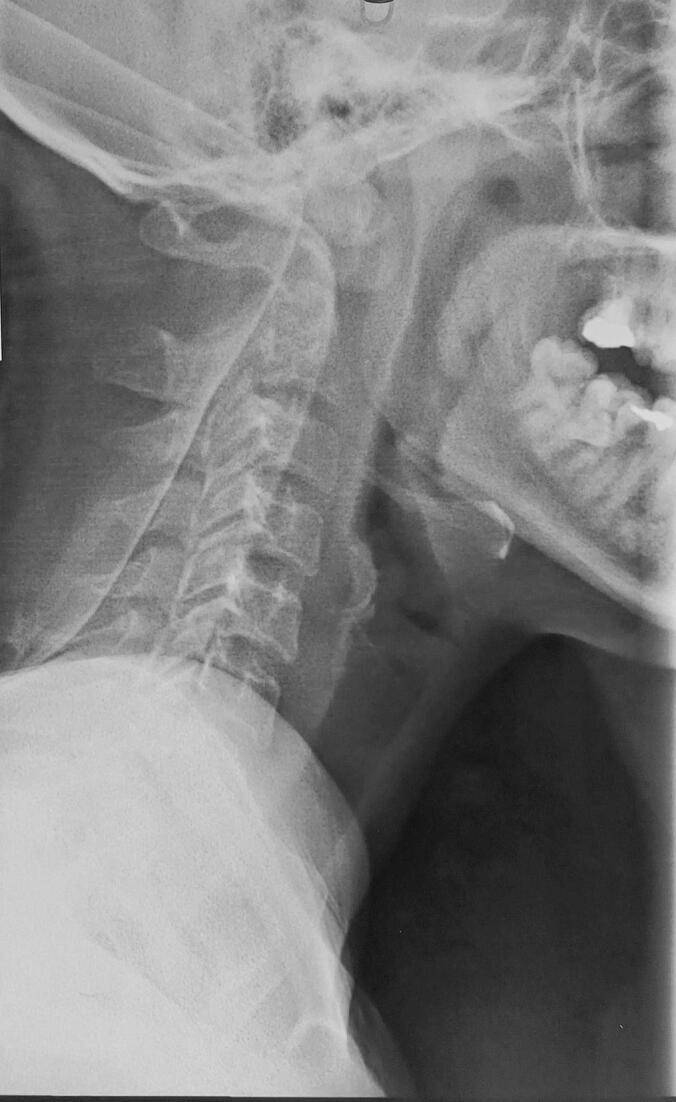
Lateral radiograph of the cervical spine showing marked atlantoaxial dislocation with anterior displacement of the atlas (C1) relative to the axis (C2). There is abnormal widening of the atlantodental interval, suggesting atlantoaxial instability. The alignment of the subaxial cervical vertebrae appears preserved.
